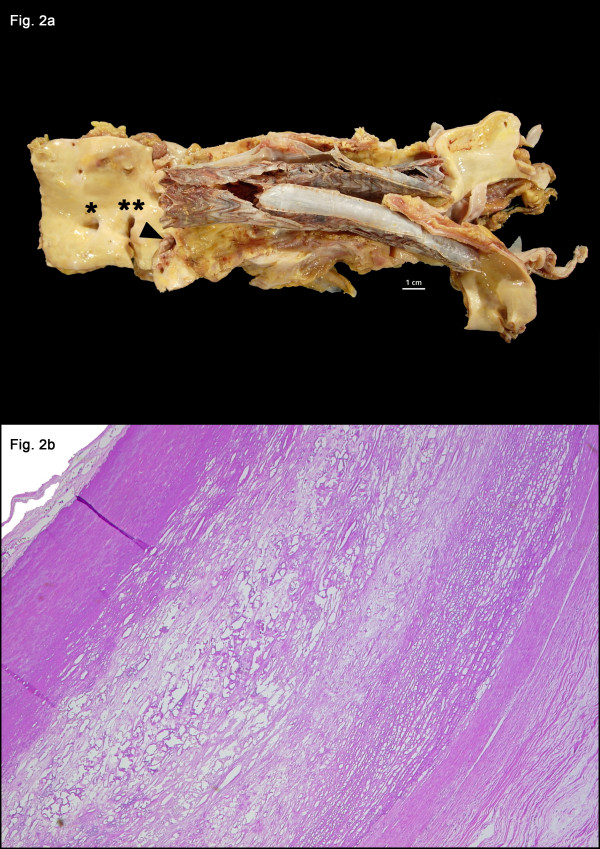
A series of abdominal computed tomography (CT) scan studies from the patient, the first at the time of diagnosis one week before endovascular aortic repair (EVAR) and regular follow-up computed tomography (CT) scans one week after EVAR and after three, six, 12 and 15 months. The initial intramural hematoma and soft tissue stranding has completely disappeared three months after EVAR and no signs of persistent or relapsed infection are detectable on follow up scans. Three months after discontinuation of antibiotic therapy (15 month after EVAR) there are no signs of relapse.
